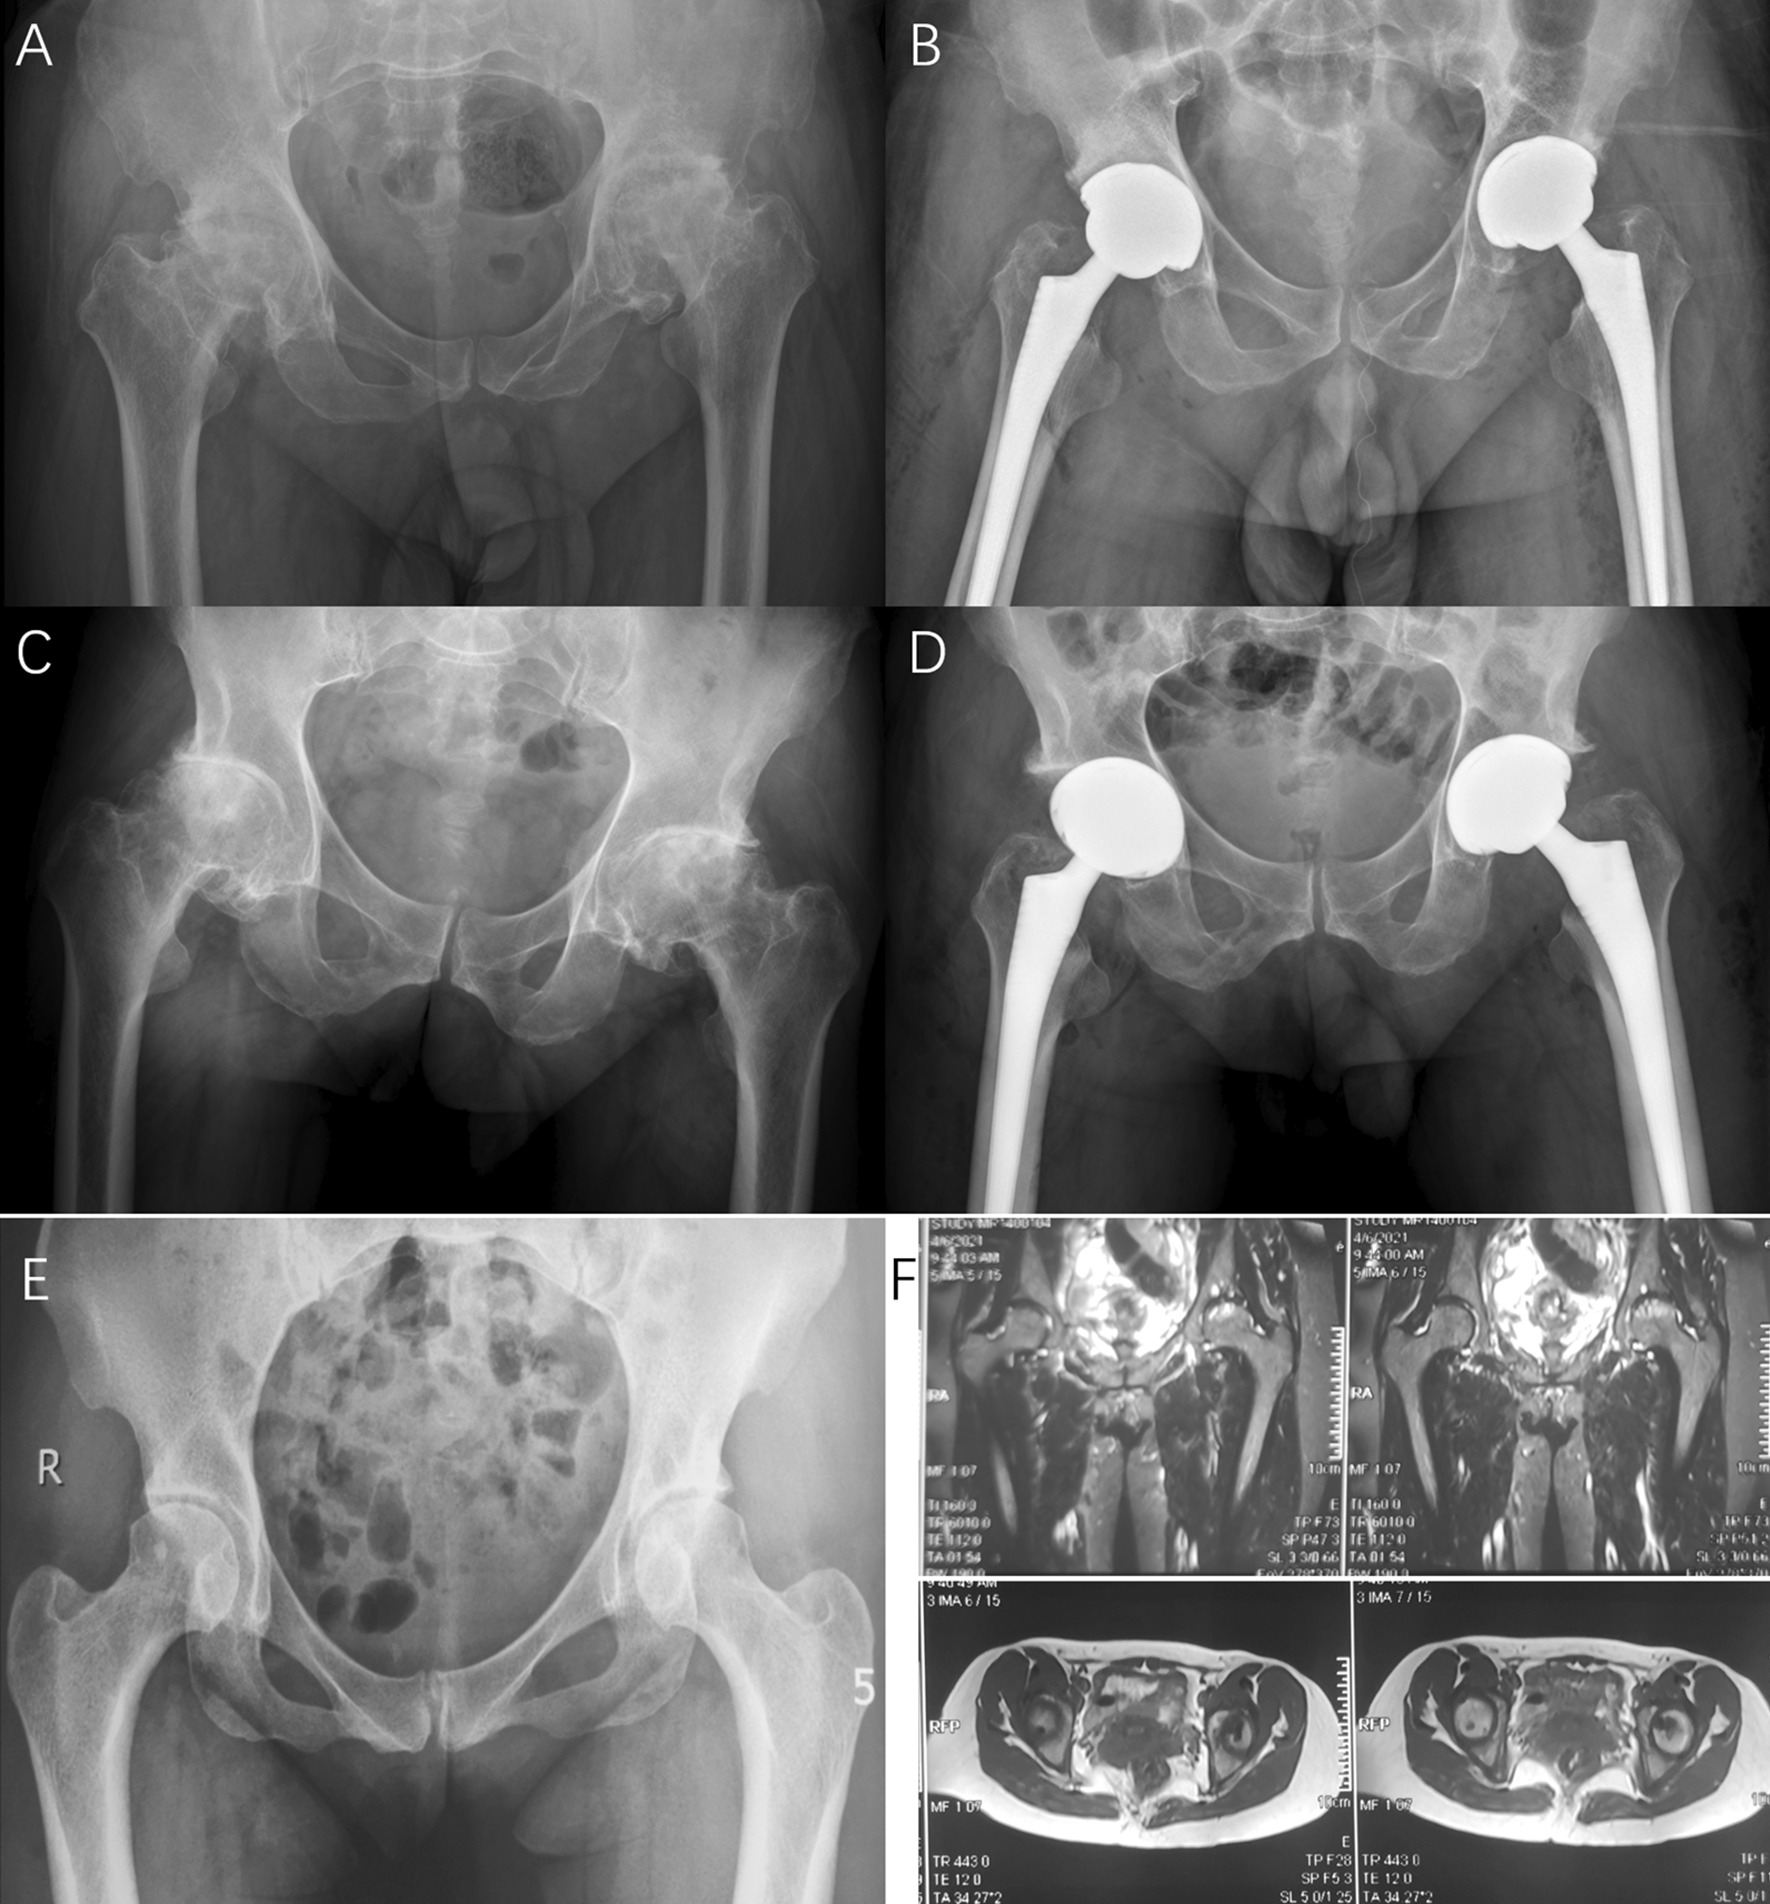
A, B the pre-operation and post-operation radiographs of IV3. C, D the pre-operation and post-operation radiographs of IV7. The pre-operation radiographs of both patients (IV3 and IV7) showed severe collapse and deformity of the femoral heads with hip joint space narrowing and osteoarthritis. E The radiograph of V2 showed the surface of the femoral head is smooth with no joint space narrowing, but there is localized increasing of bone density and sclerosis at weight-bearing region of the left femoral head. F MRI of V2 showed the crescent-shaped hyperintensity region on T2W and lower and uneven signal of necrosis region on T1W in the left femoral head
